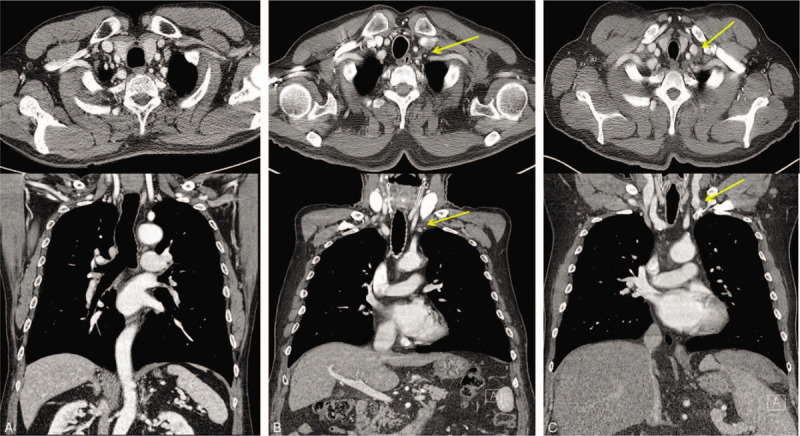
Measurement of the maximum diameter of the distal thoracic duct on the axial dimension of chest computed tomography (CT). The distal thoracic ducts noted on the axial and coronal dimensions of contrast enhanced chest CT, as indicated by yellow arrows, and the maximum diameter on axial dimension was selected. The axial and coronal images of distal thoracic duct dilatation grade 0 (A), 1 (B), and 2 (C).
